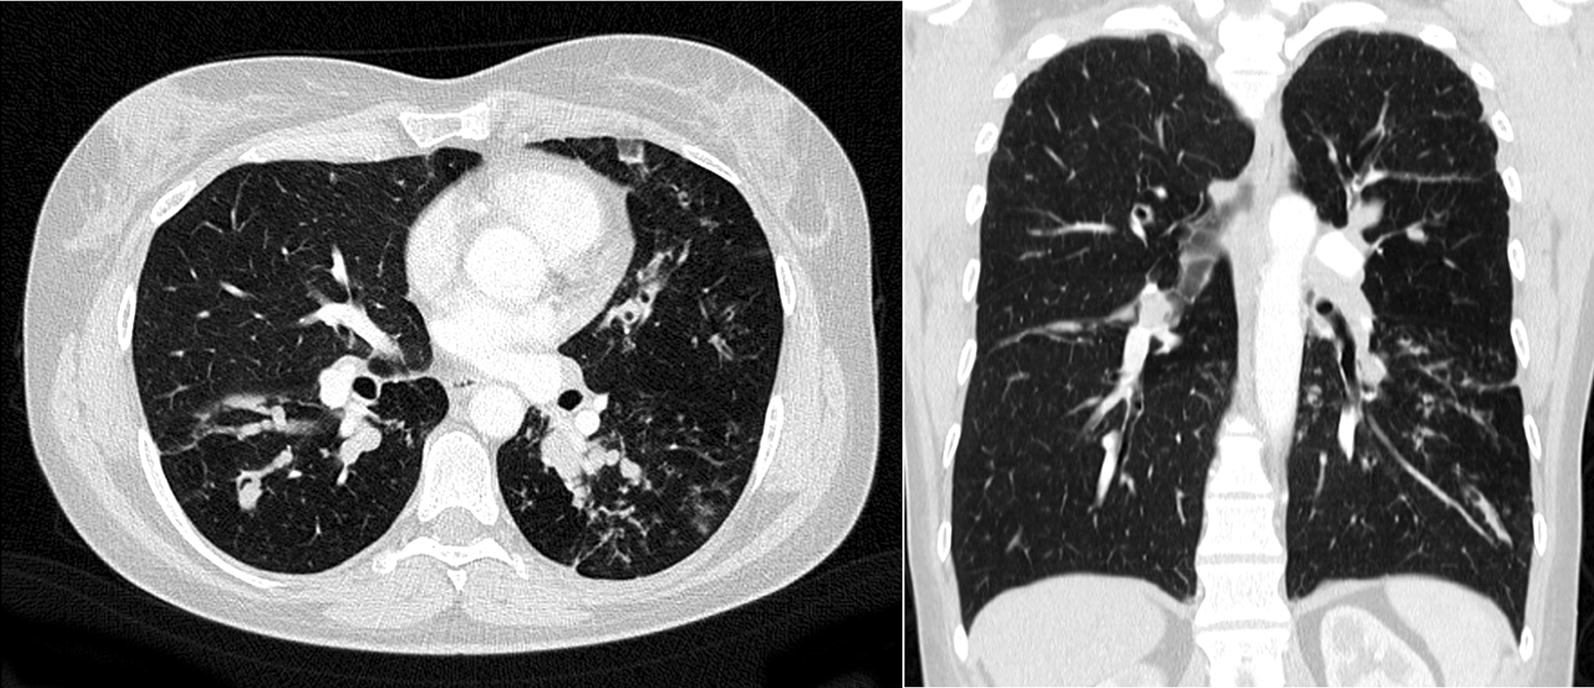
Chest CT demonstrating pulmonary infiltrates, bipulmonary bronchiectasis and bronchial wall thickening consistent with ABPA
